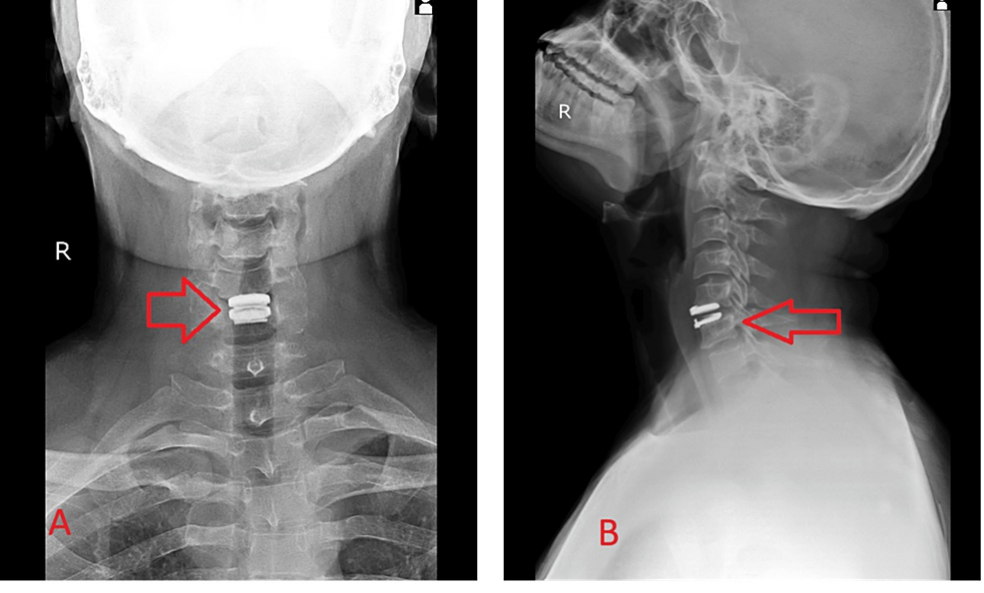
Radiographs done at three months of follow-up ((A) anteroposterior and (B) lateral views) showing a well-placed disc at the C5-C6 level with good alignment (arrows)
